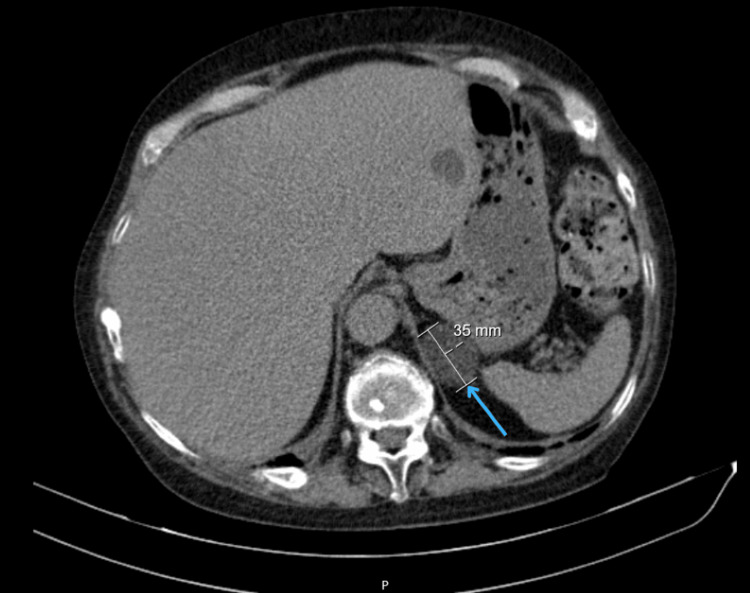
Abdominal computed tomography scan showing left adrenal mass Axial abdominal CT image demonstrating a 35 mm solid nodular lesion in the left adrenal gland (arrow), consistent with an adrenal adenoma.
